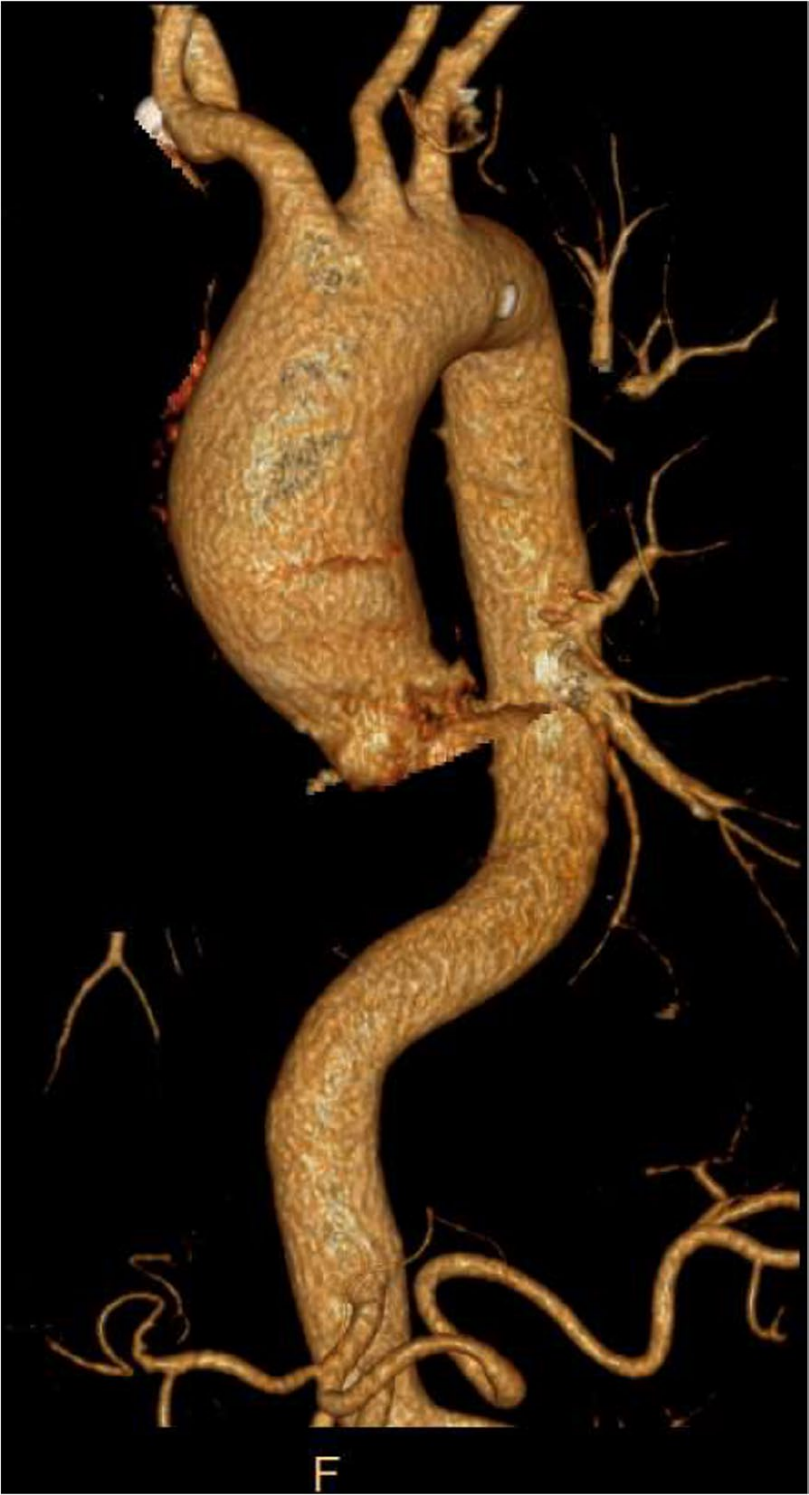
Ascending aortic aneurysm detected by imaging. Computerized tomography (CT) chest angiogram showing ascending aorta is dilated measuring maximally at 5.2 x4.6 cm cross-sectional area
